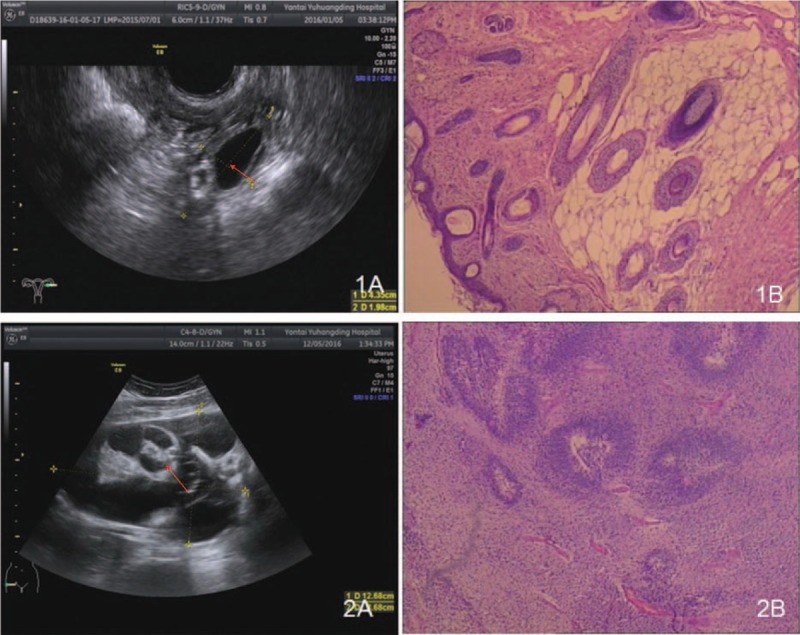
Patient 1: (1A) Ultrasound detected a hyperechoic nodule in the left ovary, with a size of approximately 1.4 × 1.2 cm^2^ (red arrow) (1B) The pathological examination revealed a cystic-solid nodule, with a wall thickness of 0.2–0.3 cm.
